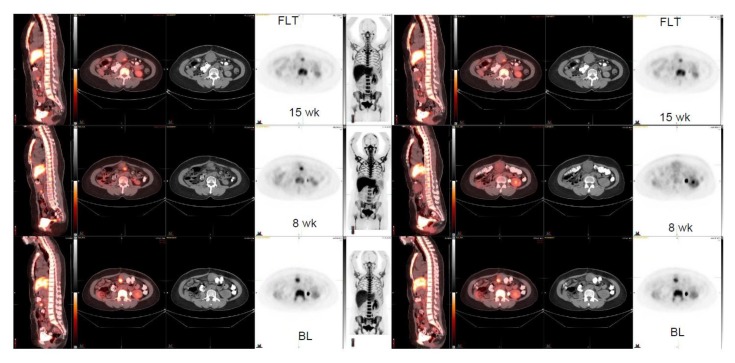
Liposarcoma. FLT-study at baseline, at 8, and at 15 weeks (3 rows). Anterior peritoneal mass is seen; the tumor consists of three components, which are in connection. The biggest tumor changes on CT as follows: 4.1 cm × 3.2 cm ->5.5 cm × 4.1 cm->5.6cm × 5.2 cm. FLT-uptakes changes in the most active site as follows: SUVmax 5.8 ->4.2 -> 3.5.
